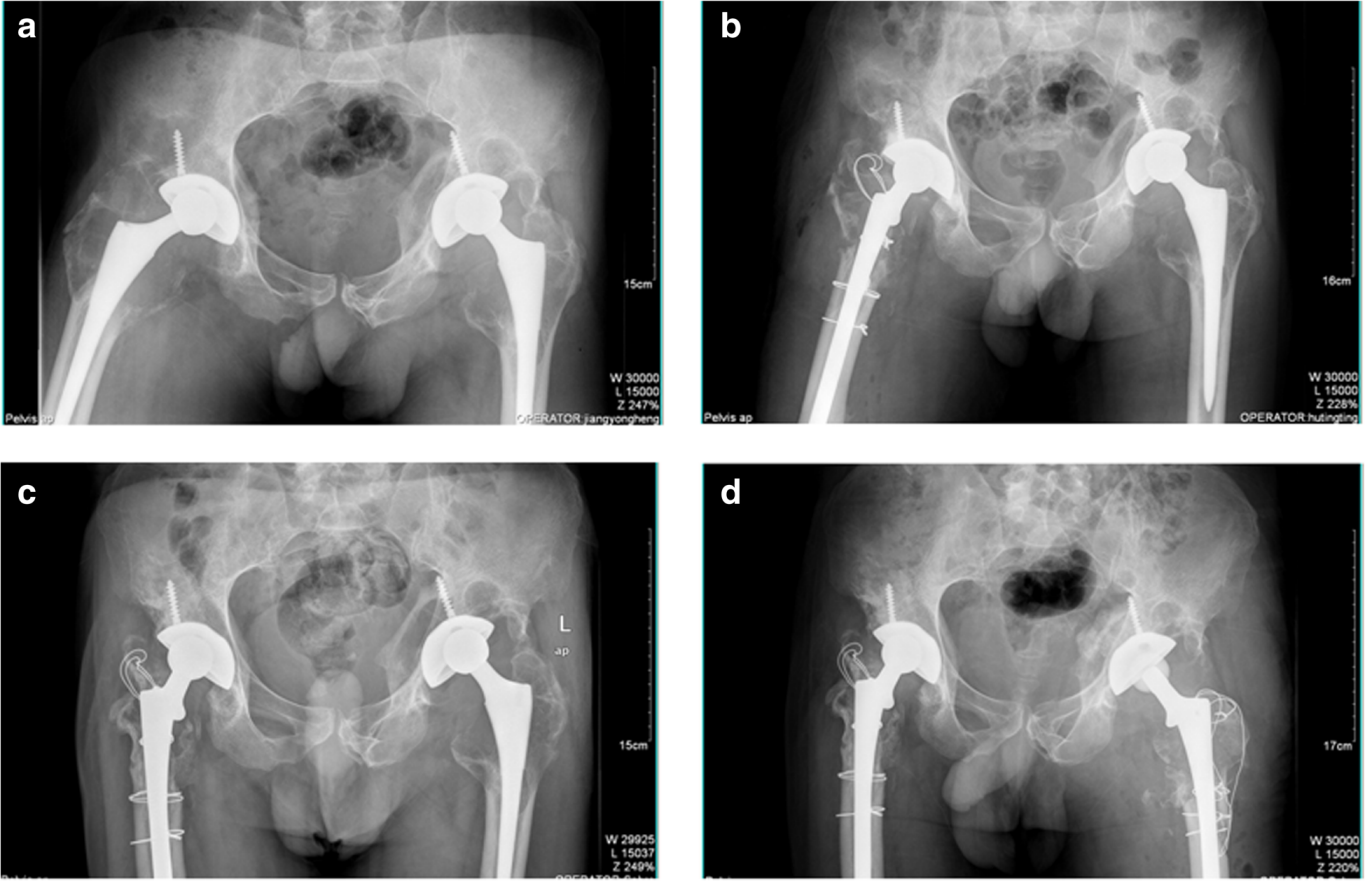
a: Pre-operative anteroposterior (AP) radiographs taken in June 2011. The patient had undergone bilateral total hip arthroplasty more than 10 years prior. The X-rays revealed wear of the acetabular linings of both hips, and severe osteolysis of both proximal femora and acetabula. b: Radiograph taken after revision of the right hip in 2011. c: Pre-operative AP radiograph taken in 2013. d: Radiograph taken after revision of the left hip in 2013
